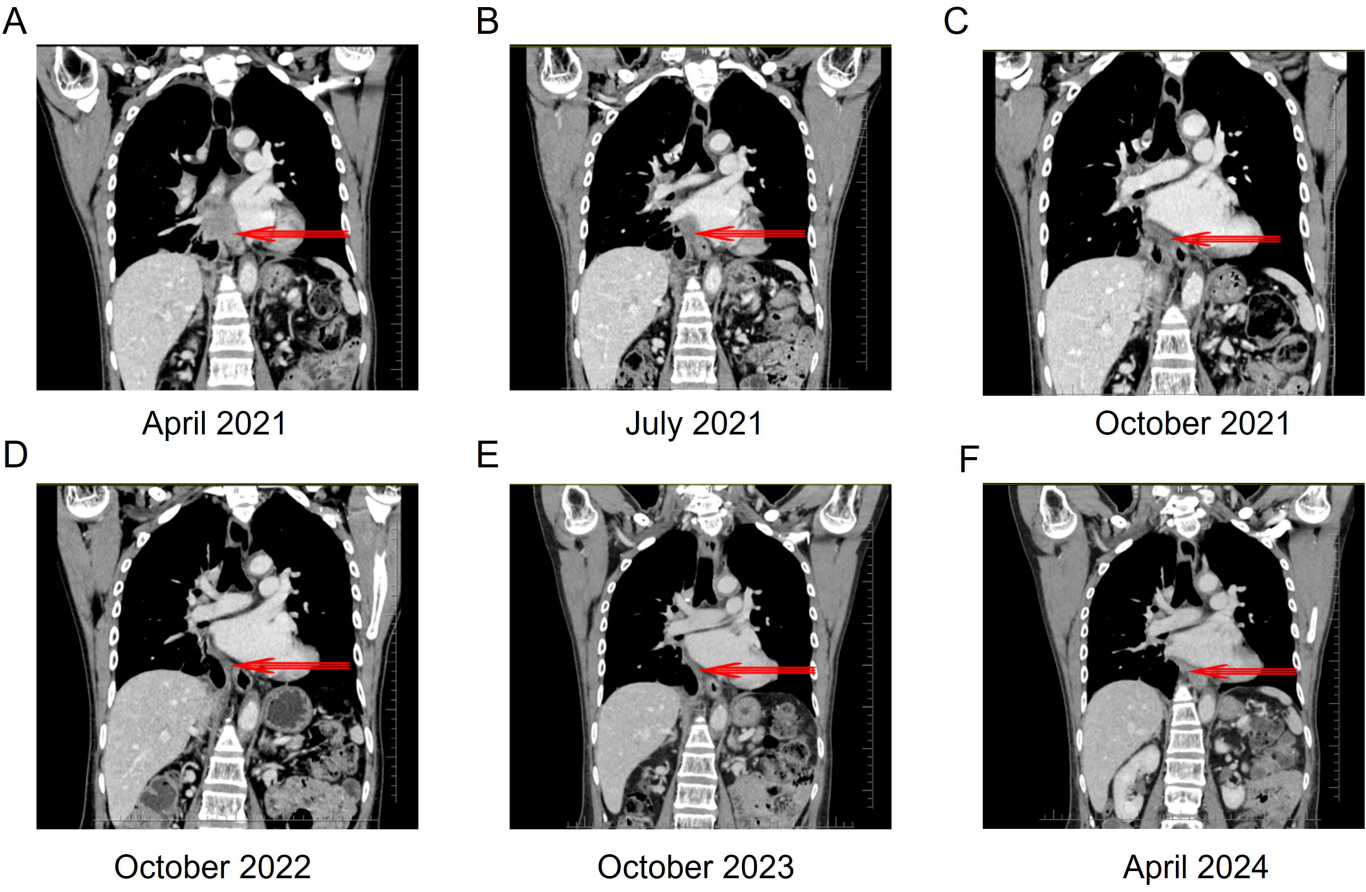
Thoracoabdominal computed tomography scan of the patient before and after ensartinib treatment. (A) Thoracoabdominal CT after 3 cycles of chemotherapy. (B) Thoracoabdominal CT after 3 months of ensartinib treatment: PR. (C) Thoracoabdominal CT after 6 months of ensartinib treatment: SD. (D) Thoracoabdominal CT after 18 months of ensartinib treatment. (E) Thoracoabdominal CT after 30 months of ensartinib treatment. (F) Thoracoabdominal CT after 36 months of ensartinib treatment. CT, computed tomography; PR, partial response; SD, stable disease.
